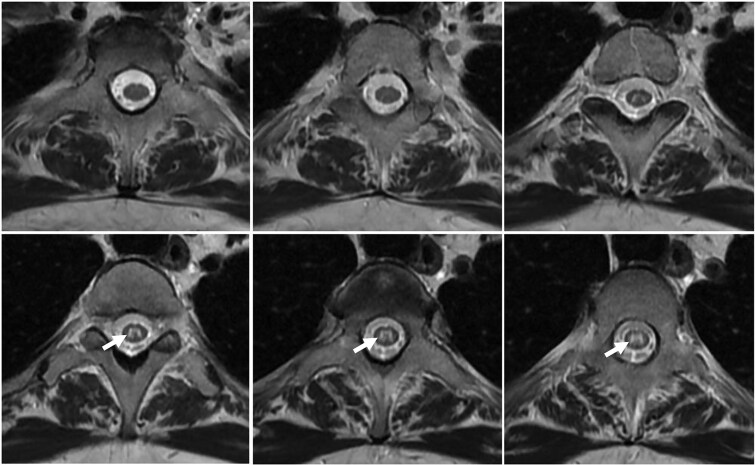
Axial T2-weighted magnetic resonance image showing the ‘H sign’.
